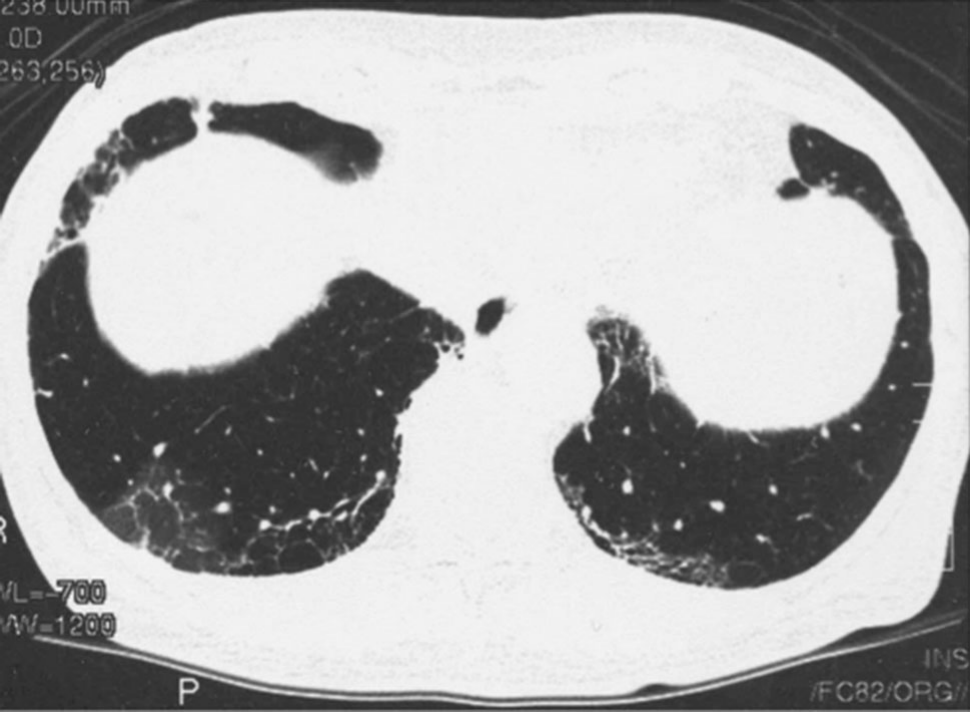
High-resolution computed tomography scan taken after 10 weeks of peginterferon-α2a treatment showing bilateral linear and lenticular interstitial opacities predominantly in the lung bases and periphery
